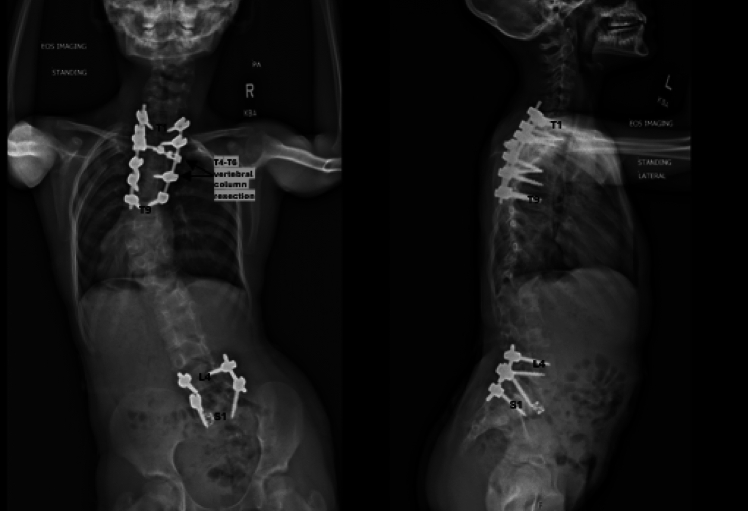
Case 2 Post-operative PA and lateral X-ray of a 9-year-old female with congenital scoliosis
